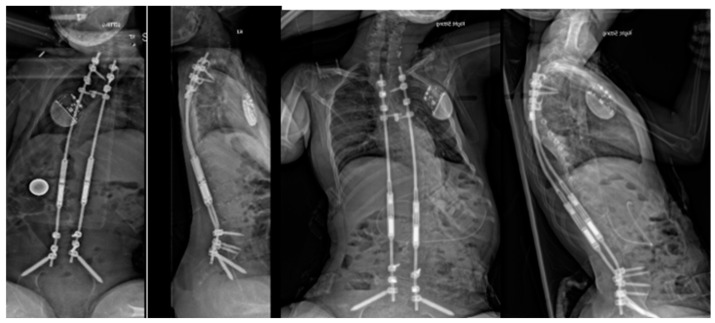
Postoperative radiographs of the same patient at 6 weeks and 3 years postimplantation of magnetically controlled growing rods with sustained curve control and excellent sitting balance.
